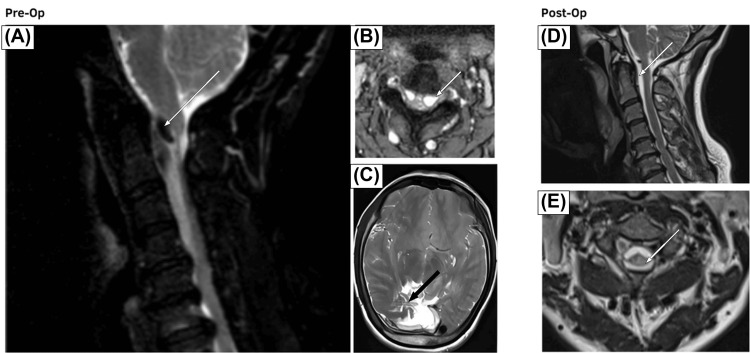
Pre- and postoperative imaging in Case 2 after revision with a programmable CERTAS valve and anti-siphon device. (A) Preoperative sagittal STIR cervical MRI showing the engorged cervical epidural venous plexus compressing the spinal cord (arrow). (B) Preoperative axial gradient-recalled echo (GRE) cervical MRI, late post–IV contrast, showing the engorged epidural venous plexus (arrow). (C) Preoperative axial T2-weighted brain MRI showing slit-like ventricles (black arrow). (D) Postoperative sagittal T2-weighted cervical MRI showing resolution of the venous engorgement with improved CSF spaces (arrow). (E) Postoperative axial T2-weighted cervical MRI showing resolution of the engorgement and cord decompression (arrow). STIR, short tau inversion recovery. CERTAS valve and anti-siphon device (Codman CERTAS® Plus Programmable Valve; Integra LifeSciences, Princeton, NJ, USA).
